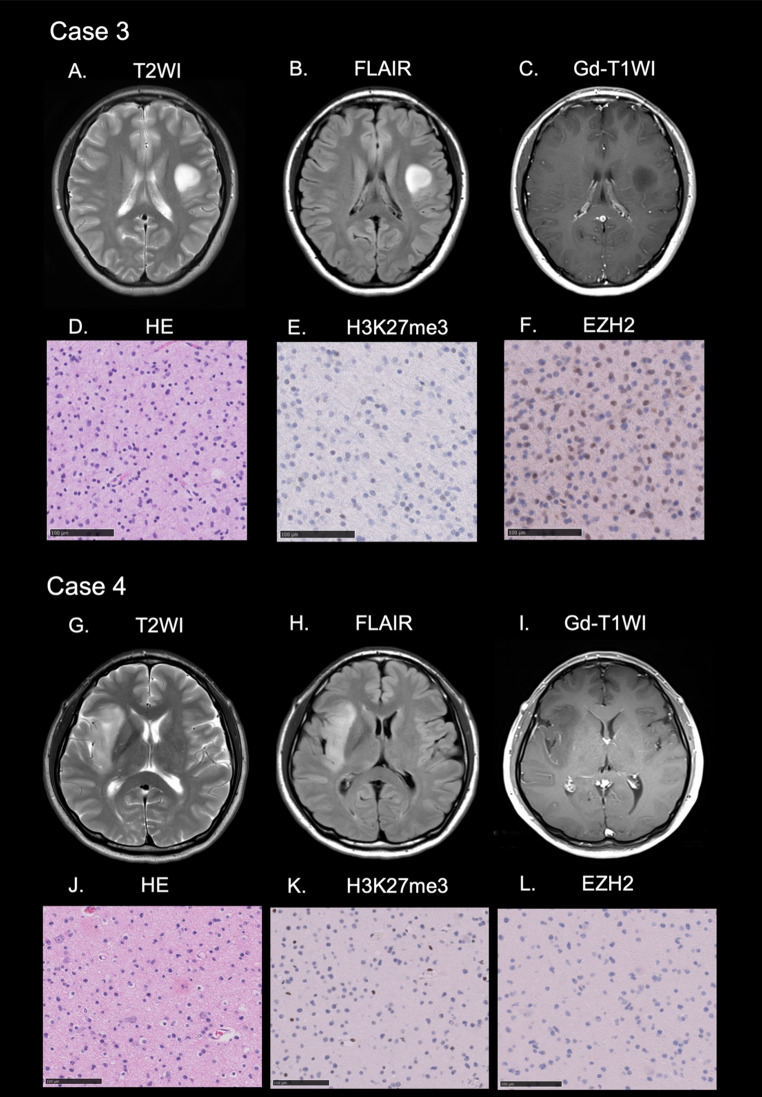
Case 3. MRI revealed a left frontal tumor with hyperintensity on T2WI (A) and FLAIR (B). The tumor displayed no contrast enhancement on post-gadolinium T1WI (C). HE revealed a diffuse astrocytic tumor (D). IHC disclosed the loss of H3K27me3 (E) and positivity for EZH2 (F). Case 4. MRI revealed a right frontal lobe and insular tumor with hyperintensity on T2WI (G) and FLAIR (H). The tumor displayed no contrast enhancement (I). HE revealed a diffuse astrocytic tumor (J). IHC disclosed the loss of H3K27me3 (K) and loss of EZH2 (L)
